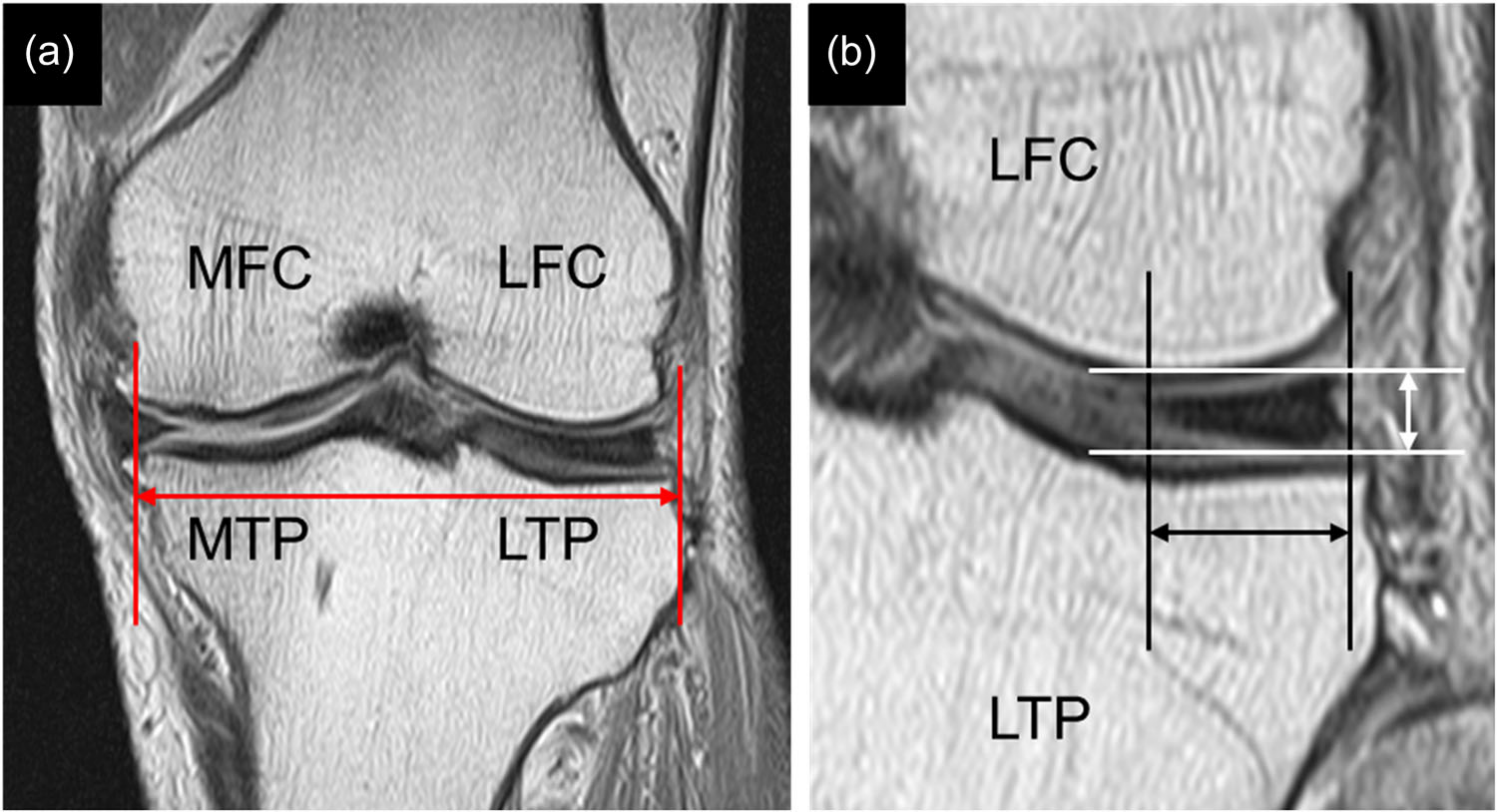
Magnetic resonance imaging‐based measurement of lateral meniscus (LM) morphology on coronal views showing the left knee. (a) The tibial width was defined as the maximum mediolateral distance across the tibia (red double arrow). (b) The LM width, the minimum width of the LM on the slice showing the most prominent meniscus body (black double arrow). LM height, measured vertically from the lowest to highest point of the meniscus (white double arrow). LFC, lateral femoral condyle; LTP, lateral tibial plateau; MFC, medial femoral condyle; MTP, medial tibial plateau.
